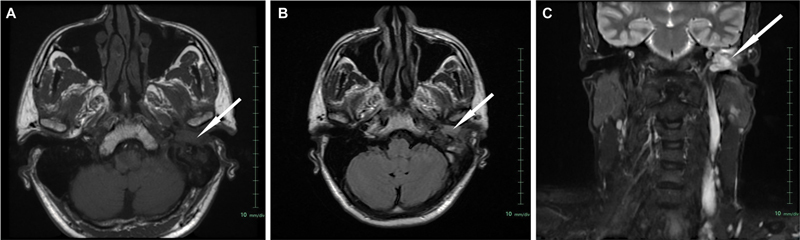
Magnetic resonance imaging showing ill-defined lobulated soft tissue lesion involving left external auditory canal and middle ear cavity and mastoid region measuring 3.1 × 2.4 × 2.5 cm (TR × AP × CC). The lesion is predominantly along the superior and posterior wall of the external auditory canal and erodes these walls. Medially it involves the middle ear cavity. There is extensive erosion of the squamous part of temporal bone with soft tissue extending into the middle ear and mastoid air cells. No obvious intracranial extension noted. Horizontal part of the facial nerve was involved. Soft tissue lesion (
